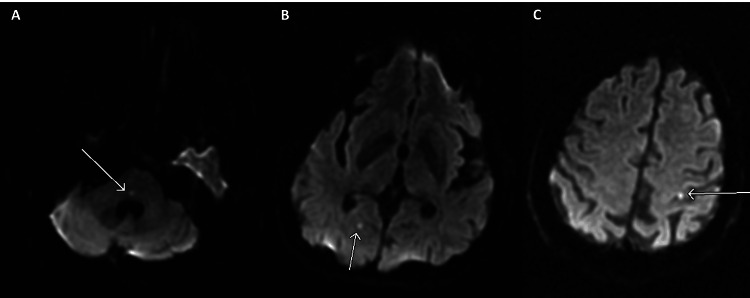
Brain MRI axial DWI: multiple acute punctuate infarcts: (A) right pons, (B) right occipital, and (C) left parietal. DWI, diffusion-weighted imaging; MRI, magnetic resonance imaging
